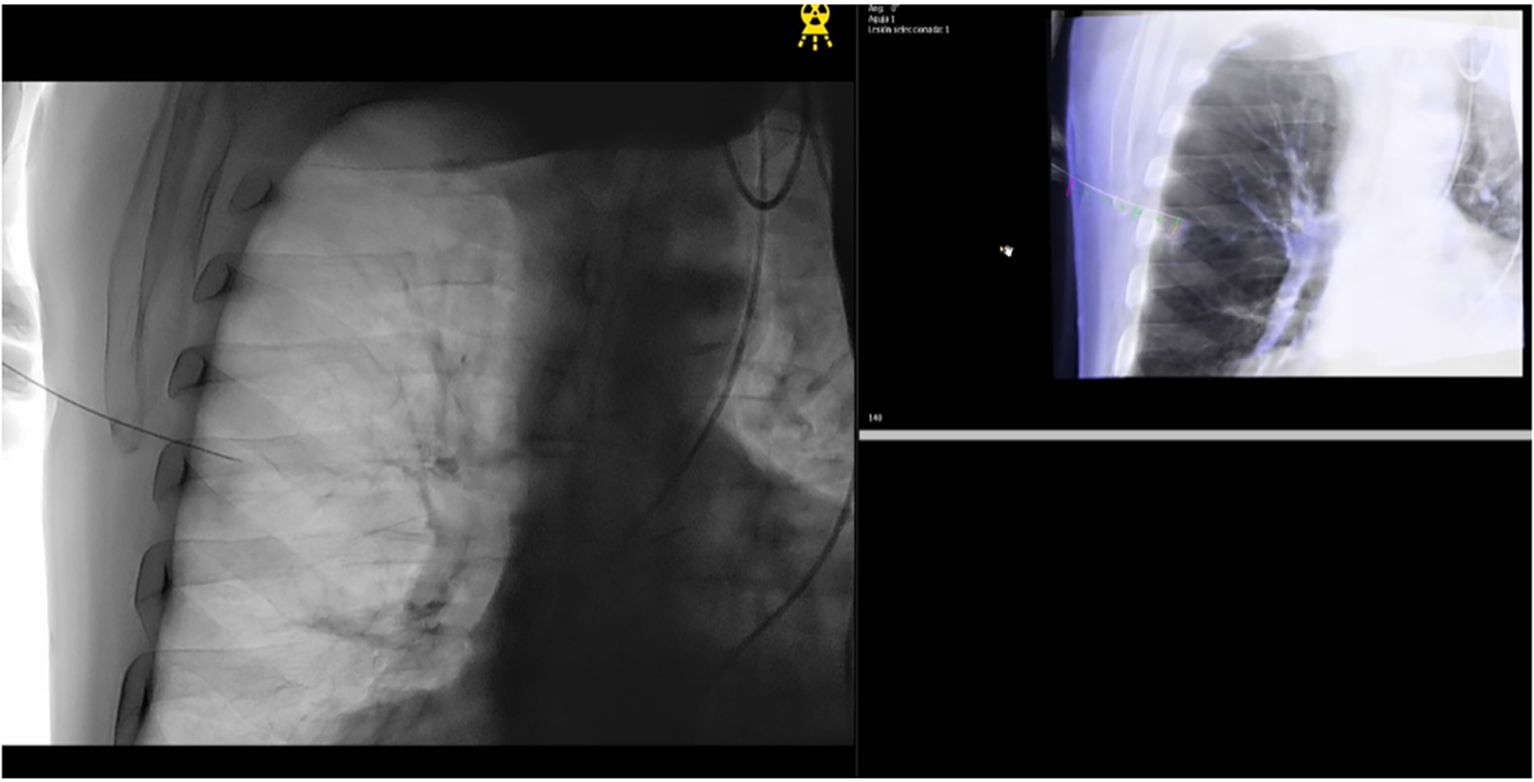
Imaging of electromagnetic navigation bronchoscopy (ENB) marking with cone beam computed tomography (CBCT) assistance.
